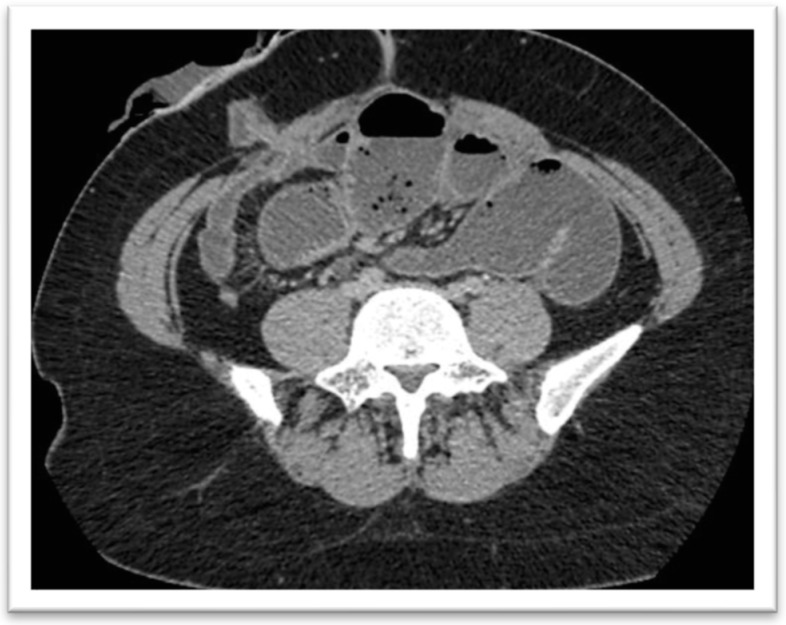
Abdominal CT scan demonstrating signs of small bowel obstruction in a patient with CD following proctocolectomy with end ileostomy.
